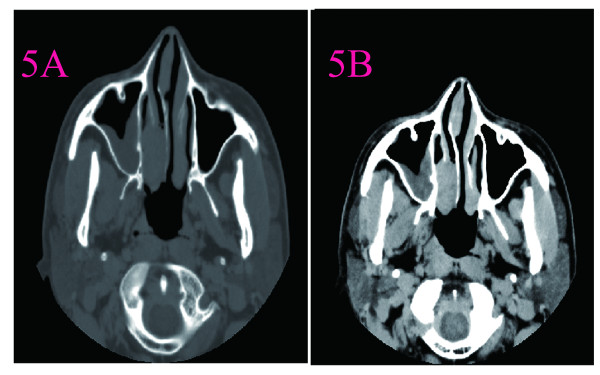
CT scans of nasal cavity in Case 4. A:CT scanning showed a 2.0 cm in diameter soft density mass that filling the left choana and extended into the nasopharynx. B: CT with contrast injection showed that the mass enhanceing inhomogeneously.
